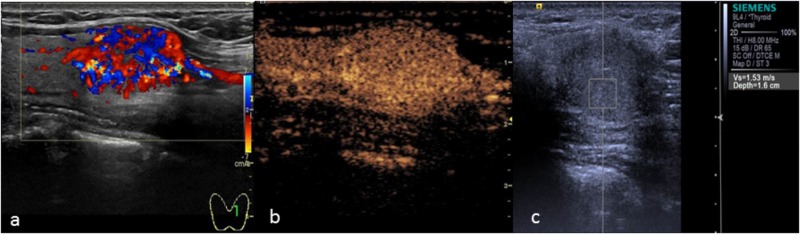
Papillary thyroid carcinoma. (a) Two-dimensional ultrasound showing a hypo-echoic nodule with calcification. (b) CEUS showing heterogeneous low enhancement. (c) The SWV value was X.XX m/s.
